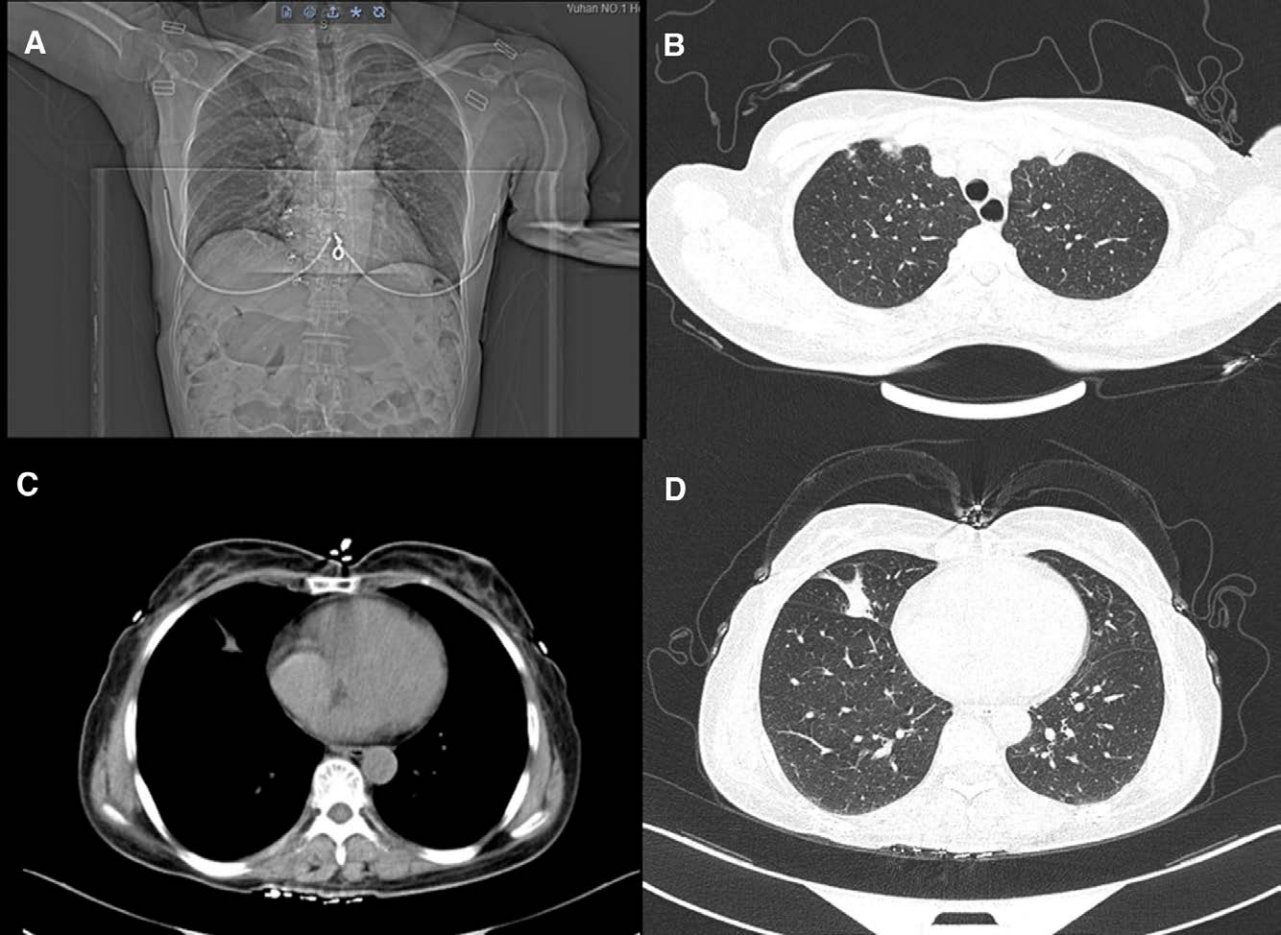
Lung CT: (A and B) Partial solid nodules in the upper lobes of both lungs, (C and D) thin-walled cavity in the right lower lobe. CT = computerized tomography.
